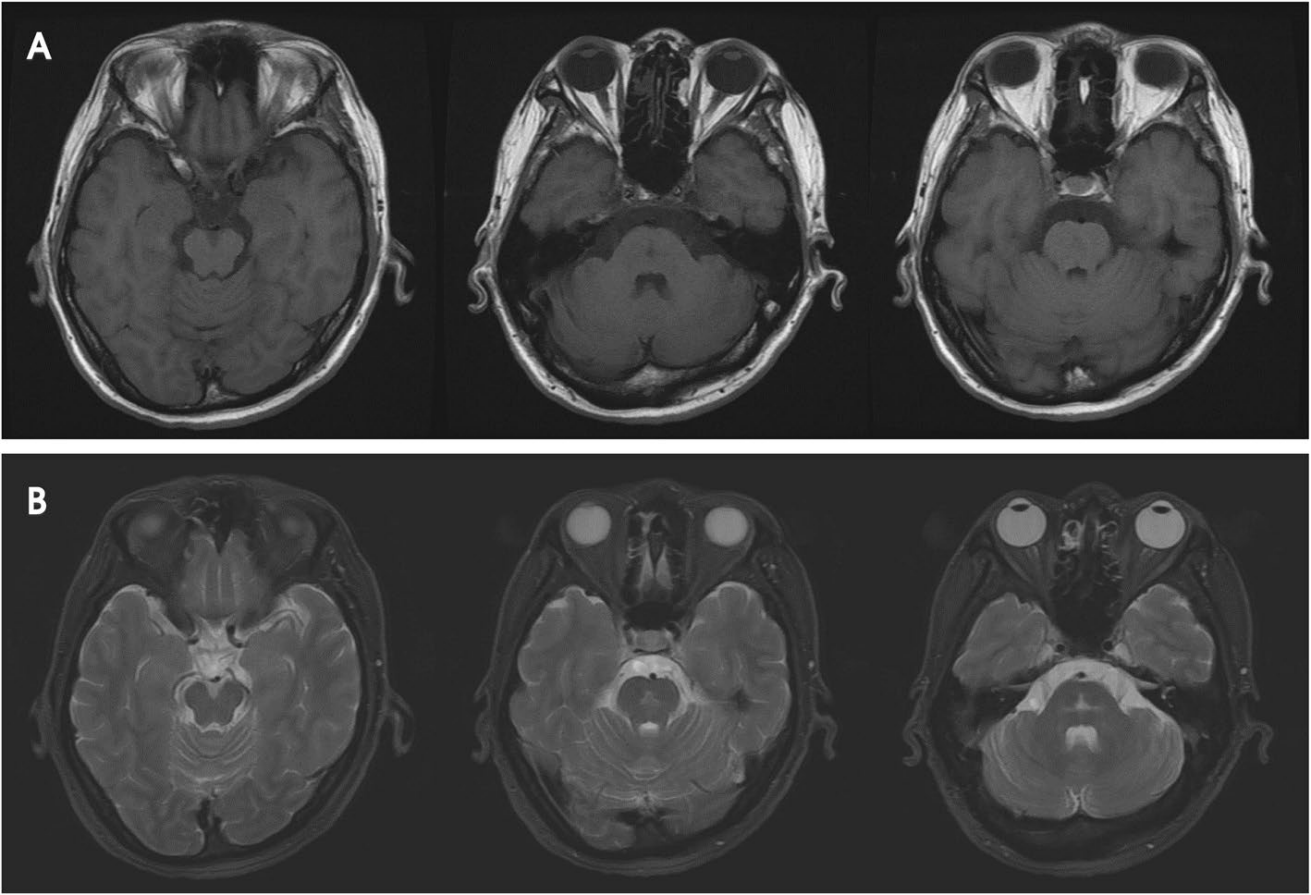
Brain MRI at 4 months after stopping tacrolimus shows partial resolution of low signal intensity lesion in the middle of pontine tegmentum on T1WI (A) and high signal intensity in the same region on T2WI (B)
